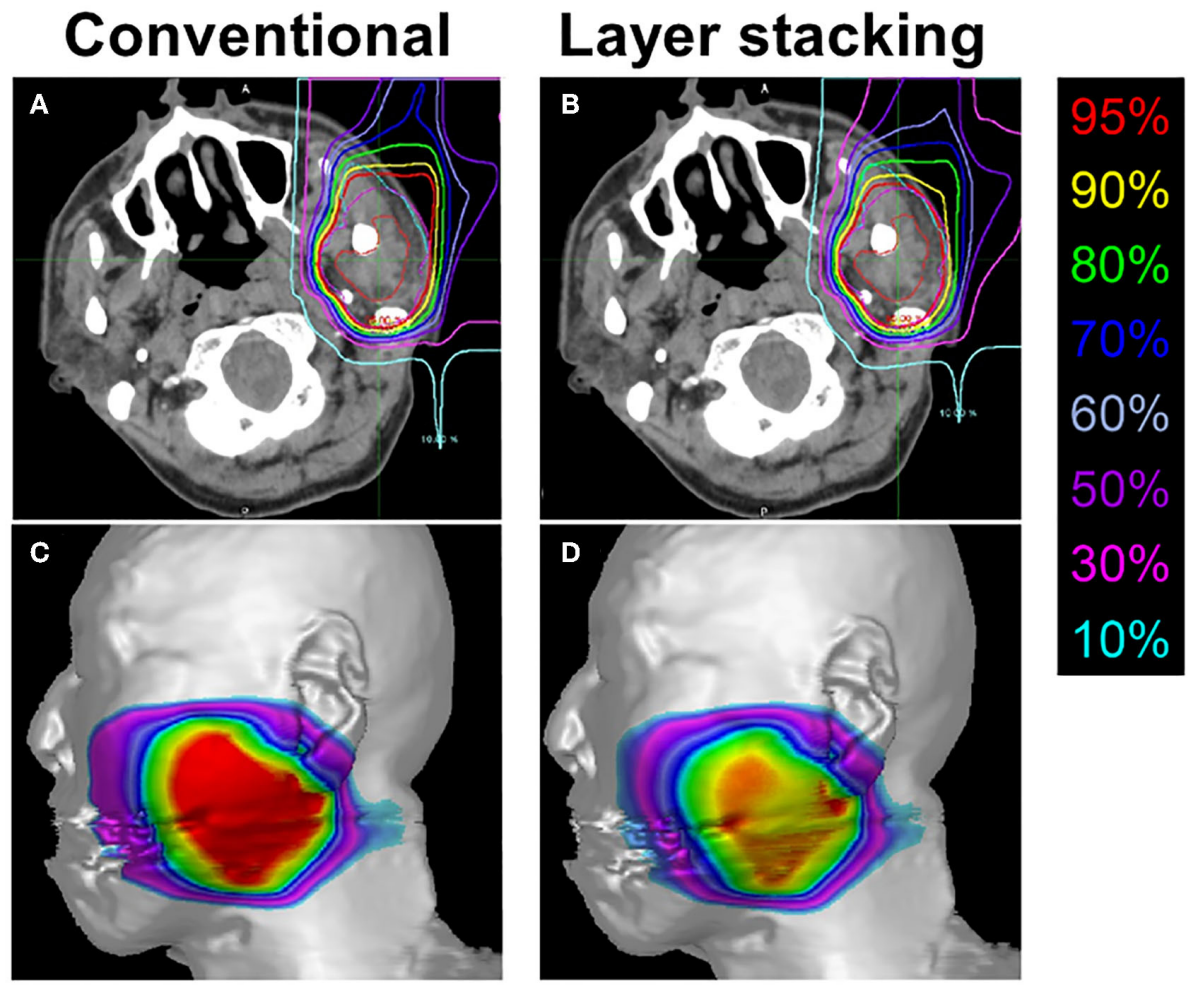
Representative treatment plans created by conventional passive irradiation and layer-stacking irradiation for the same subject. (A,B) Dose distribution in axial computed tomography images. Gross tumor volume, clinical target volume (CTV) 1, and CTV2 are indicated in red, cyan, and magenta, respectively; (C,D) dose-surface area model for the skin.
